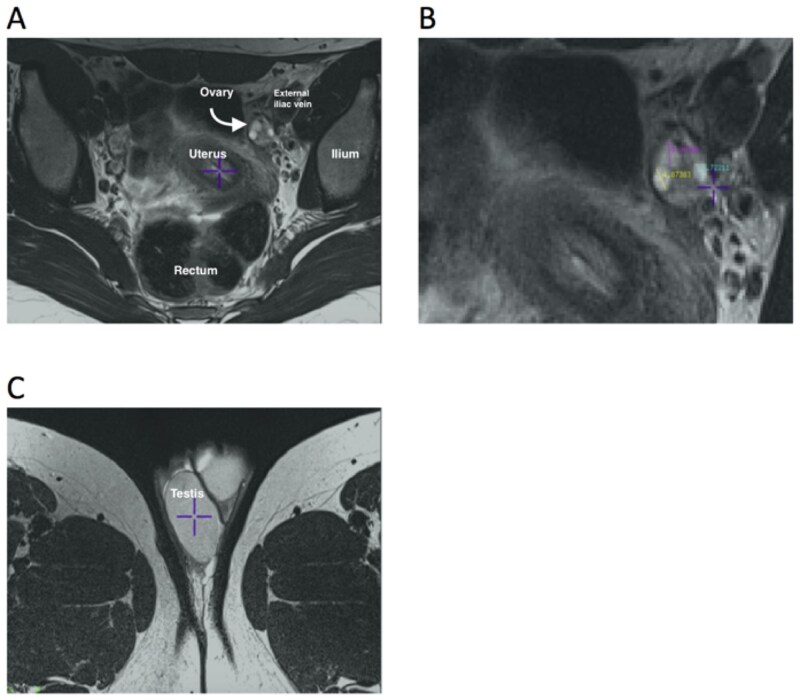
Examples of MRIs of the gonads from T2-weighted images acquired in Project Ice Storm. (A) T2-weighted image of the female pelvic region in axial view. (B) A close up of the left ovary showing three follicles. (C) T2-weighted image of the left testicle (at the crosshair) in axial view.
